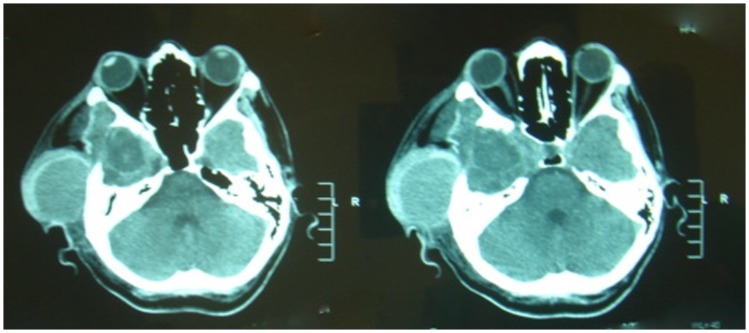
CT scan showing superficial temporal artery pseudoaneurysm.
